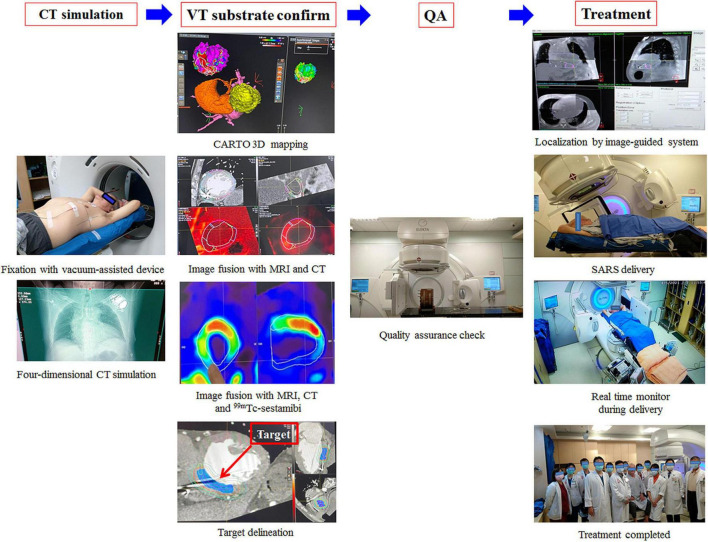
Multimodal imaging combined with scar imaging, including the CARTO mapping system (Biosense, Diamond Bar, CA), CT, MRI and ^99^*^m^*Tc-sestamibi MPI, was used offline to delineate a target for ablation. A plan was developed in the Pinnacle3 planning system (version 9.8.1, Philips Medical Systems, Madison, WI, USA). One day before treatment, quality assurance was performed. On the day of treatment, the patient was immobilized on the vacuum device, and cone-beam computed tomography (CBCT) was used for patient setup and target localization. The treatment unit was aligned with the patient, and a dose of 12 Gy was delivered precisely by volumetric-modulated arc therapy (VMAT) through a linear accelerator machine (Versa HDTM, Elekta, Crawley, West Sussex, UK) with 6 MV photons.
